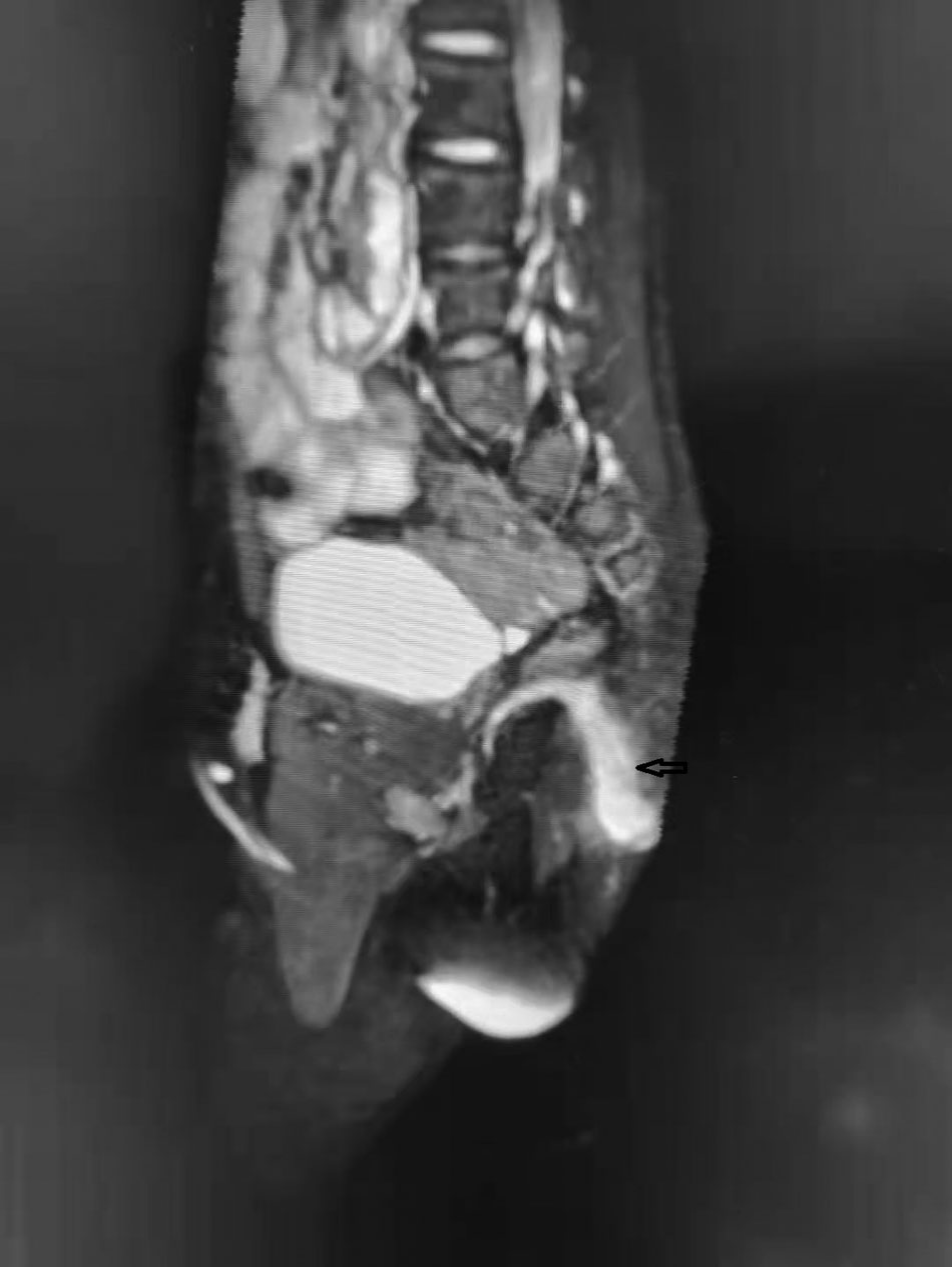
Sagittal T2-weighted perineum MRI shows a 25- mm anal fistula (arrow).
